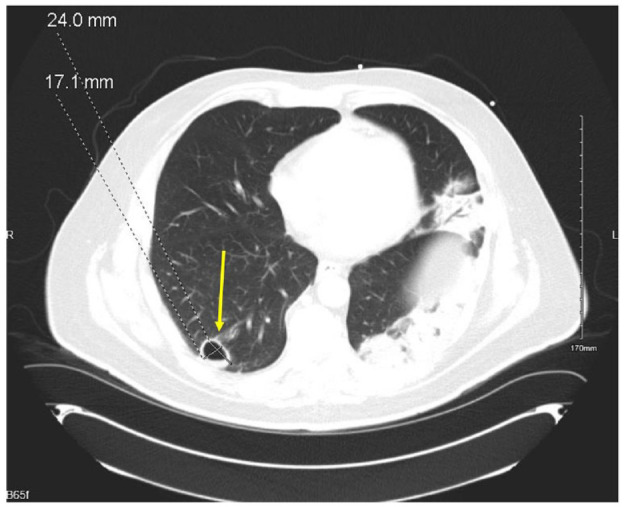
Chest x-ray revealing multifocal pneumonia.
